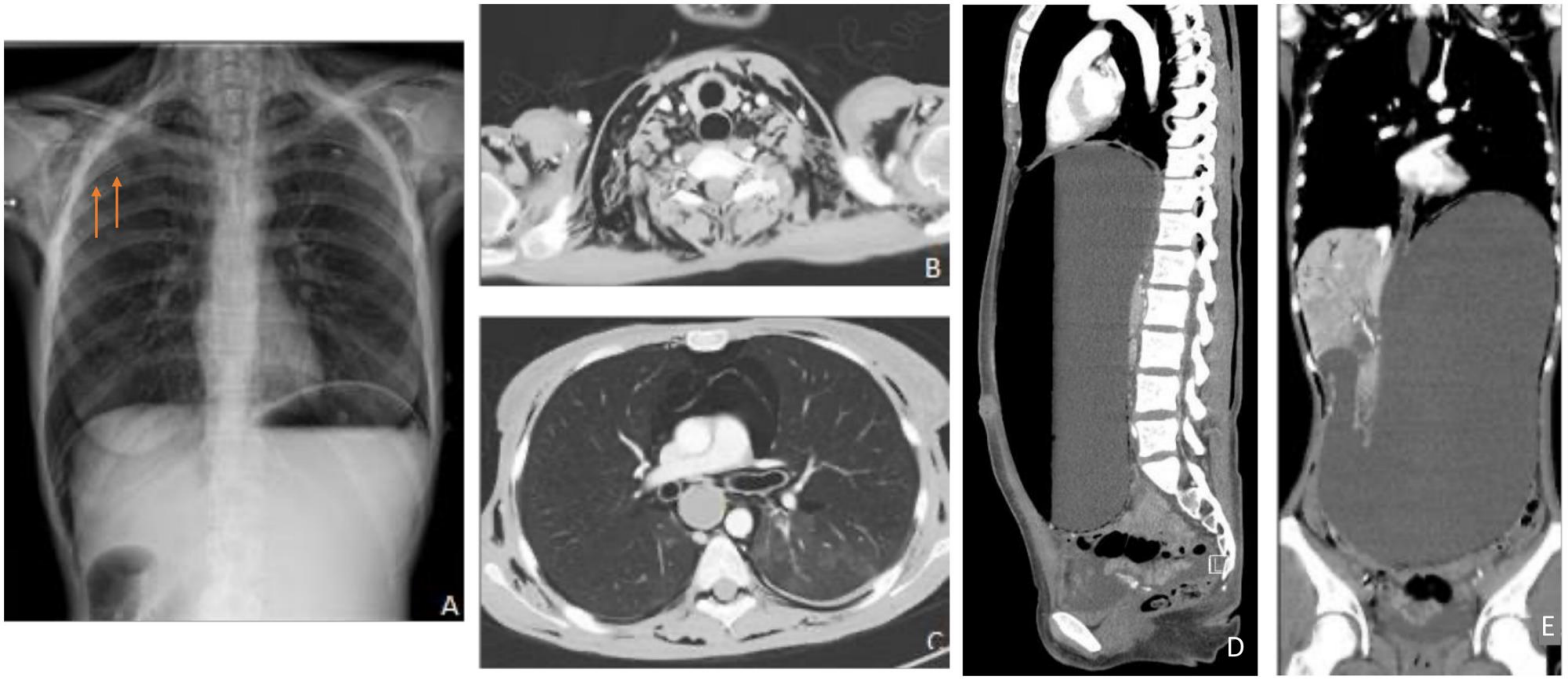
(A) Chest X-ray showing subcutaneous emphysema and a pneumothorax (indicated by orange arrows). (B) Extensive subcutaneous emphysema in the neck and mediastinum. (C) showing the pneumomediastinum. (D) and (E) sagittal and coronal reconstruction of the extensively filled stomach, with a maximum diameter of 34 cm, as well as air in the portal veins.
